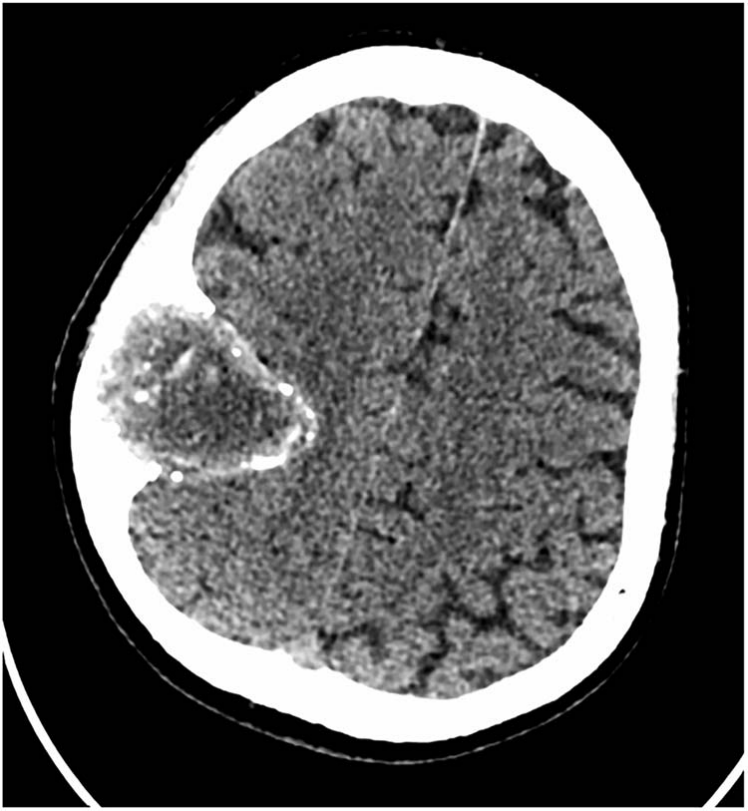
Axial CT scan showing the extra-axial lesion with surrounding bone remodelling.
